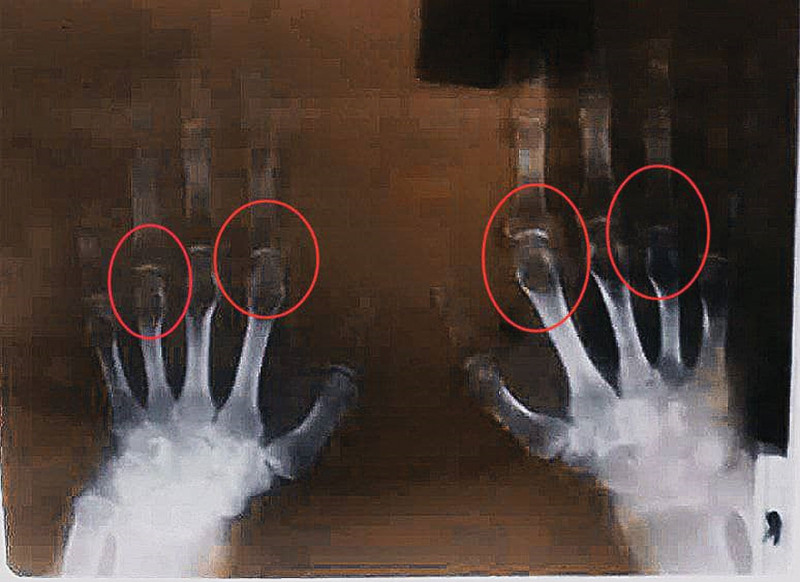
X-rays of the hands reveal bilateral and symmetrical involvement, proximal interphalangeal joint space narrowing, metacarpophalangeal joint space narrowing, and metacarpophalangeal joint osteopenia (red circles).
